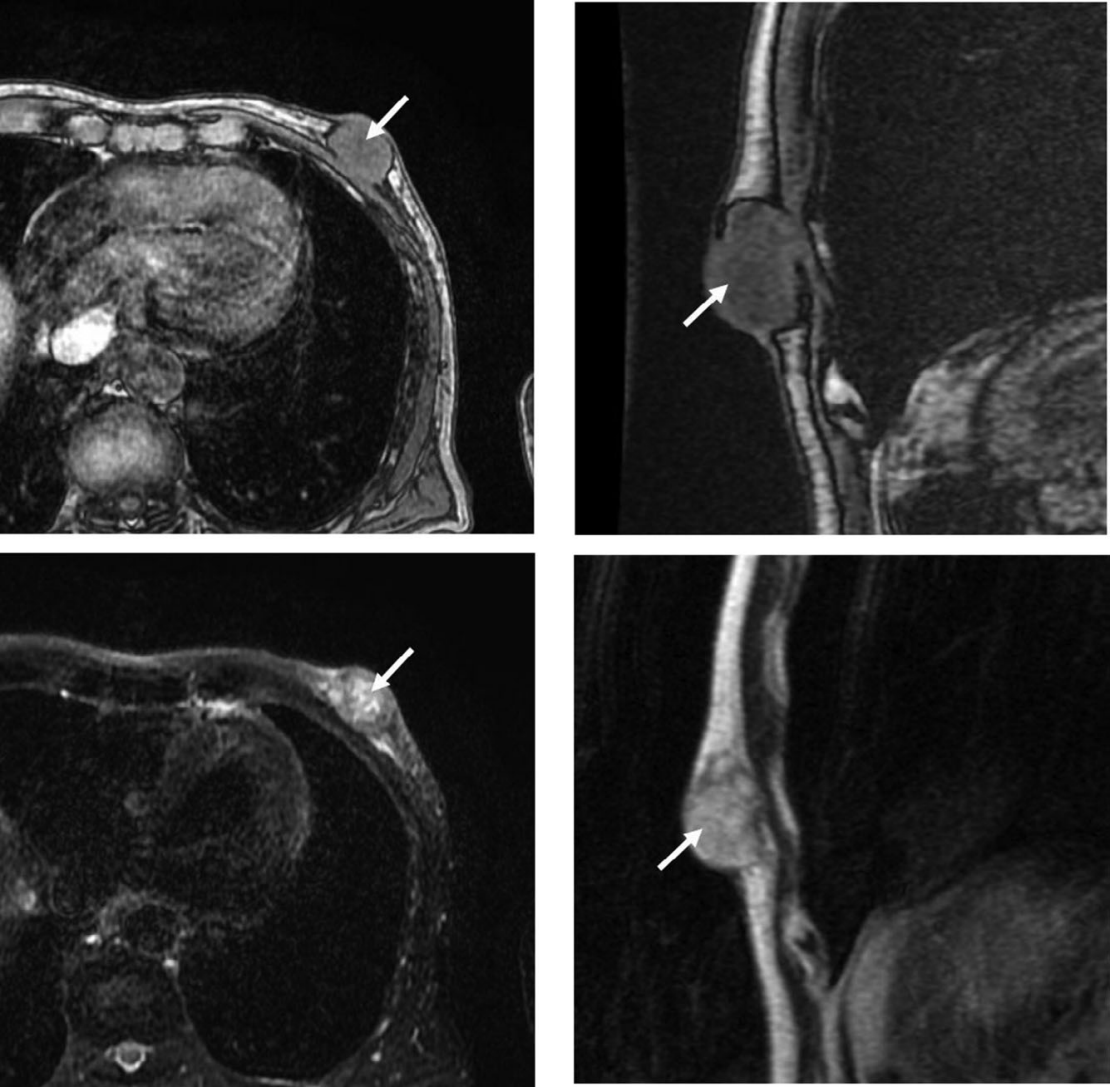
Simple magnetic resonance imaging of the left breast. Tumors with unclear boundaries and inhomogeneous content (arrows). Upper: T1‐weighted image (left: transverse section, right: sagittal section). Lower: T2‐weighted image (left: transverse section, right: sagittal section).
